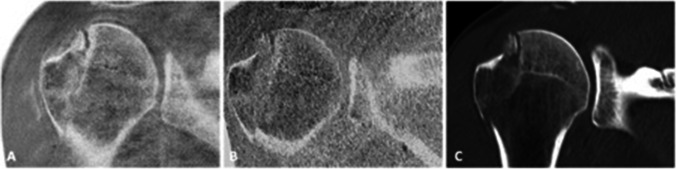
Fracture dislocation with avulsion of the greater tubercle in a 23-year-old patient admitted to the ER. The fracture was equally detectable using a FRACTURE sequence (A) as well as a T1 GRE sequence (B) compared to the conventional CT (C)
